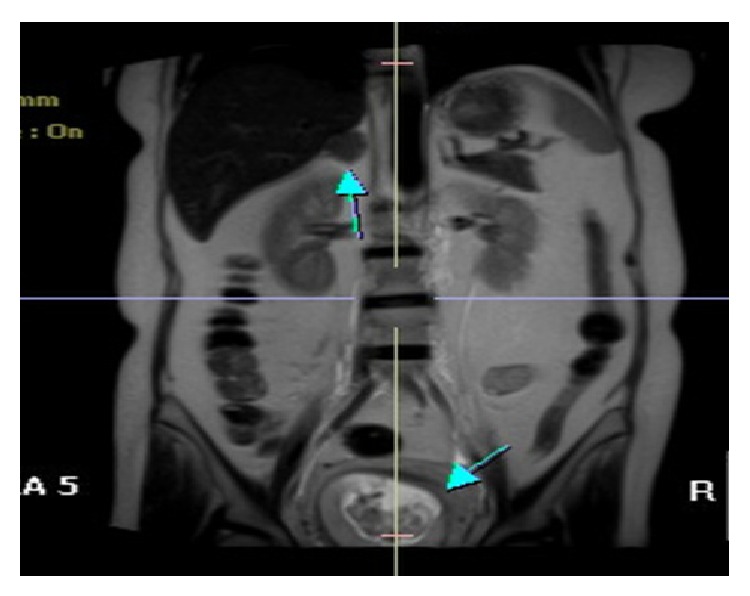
MR image of the adrenal adenoma and intrauterine fetus.
